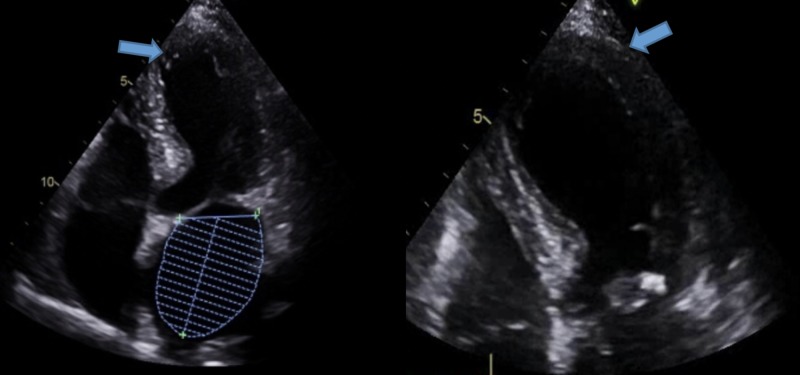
Apical four-chamber view showing apical hypokinesia and ballooning characteristic of Takotsubo cardiomyopathy. The image on the right is an expanded view of the image on the left. Both images here show the characteristic LV ballooning of the apex.
